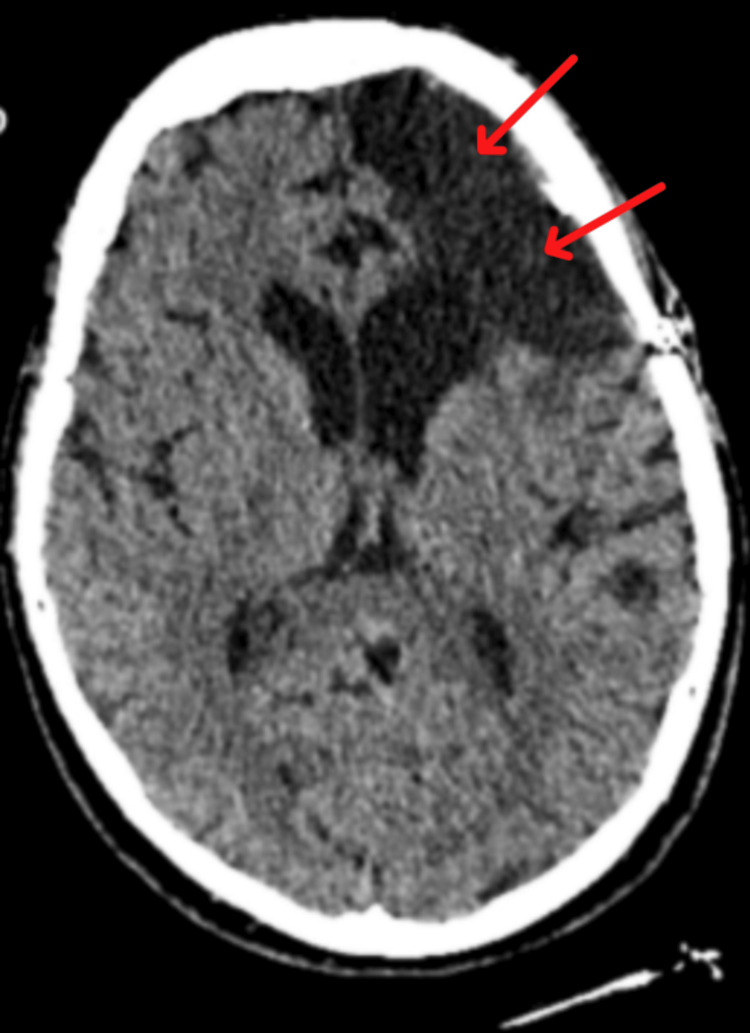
Non-contrast CT of the brain. Image demonstrating prior left frontal craniotomy changes. Red arrows indicate areas of hypodensity and volume loss consistent with post-operative encephalomalacia at the surgical margins, without evidence of acute intracranial infection.
